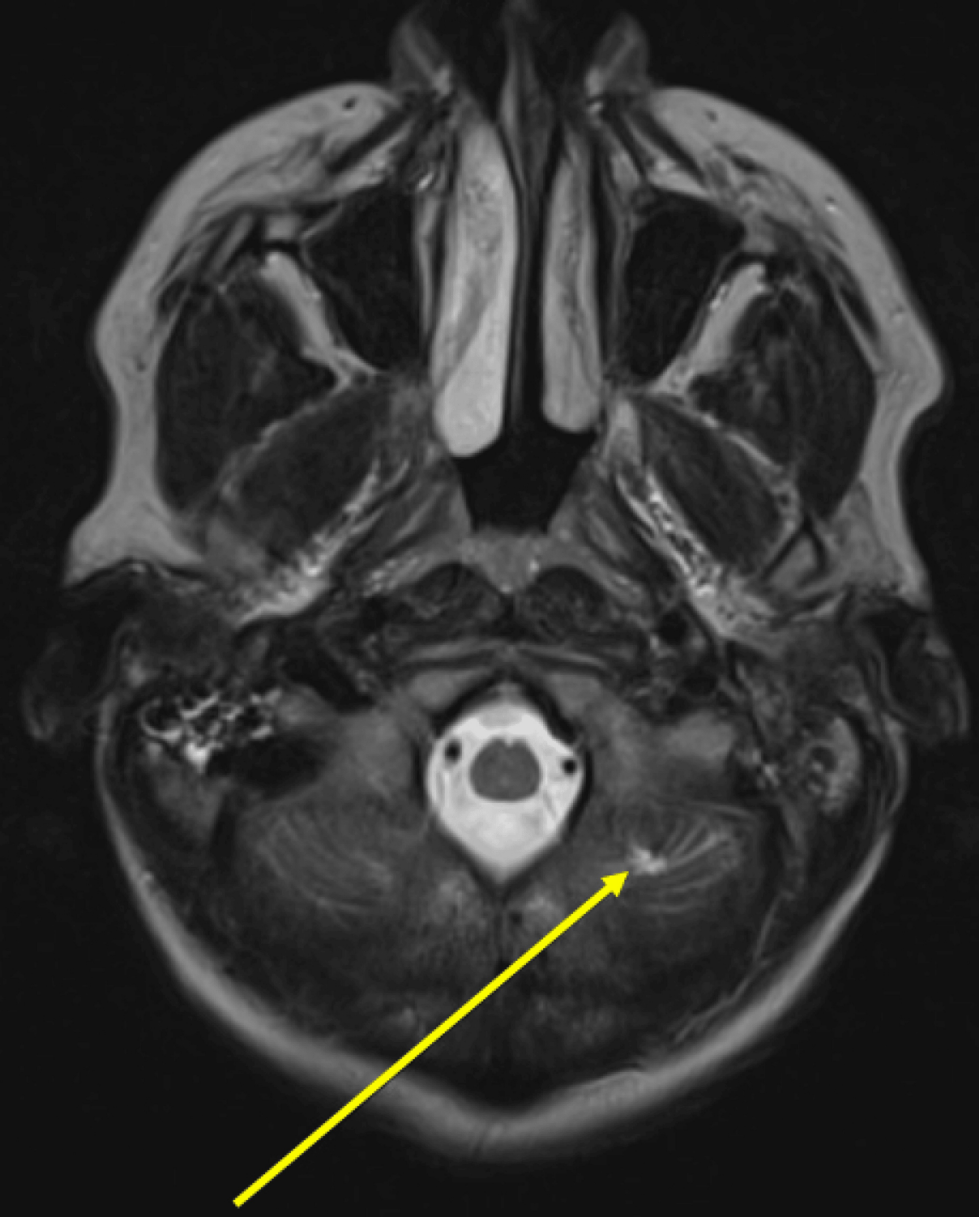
Silent cerebellar infarct discovered at age 43. Note the chronic lacunar infarct in the left cerebellar hemisphere (arrowhead) on the T2-weighted image of the brain MRI.
